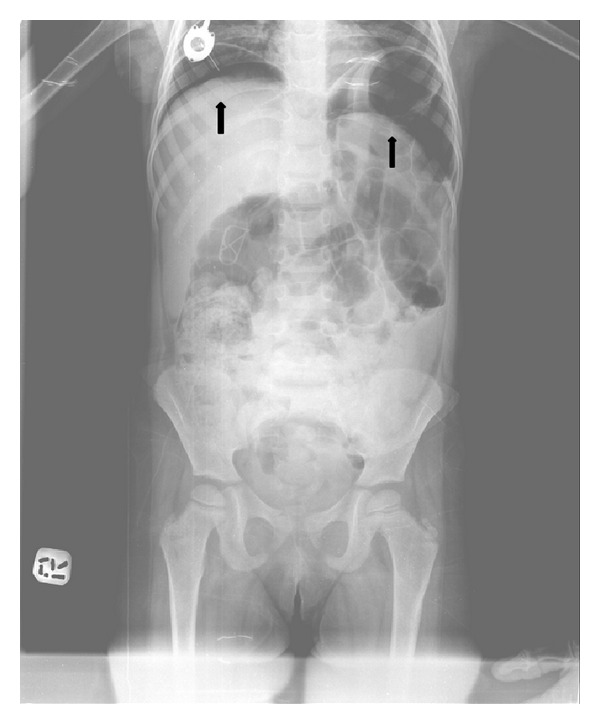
Plain abdominal radiograph shows subdiaphragmatic free air.
